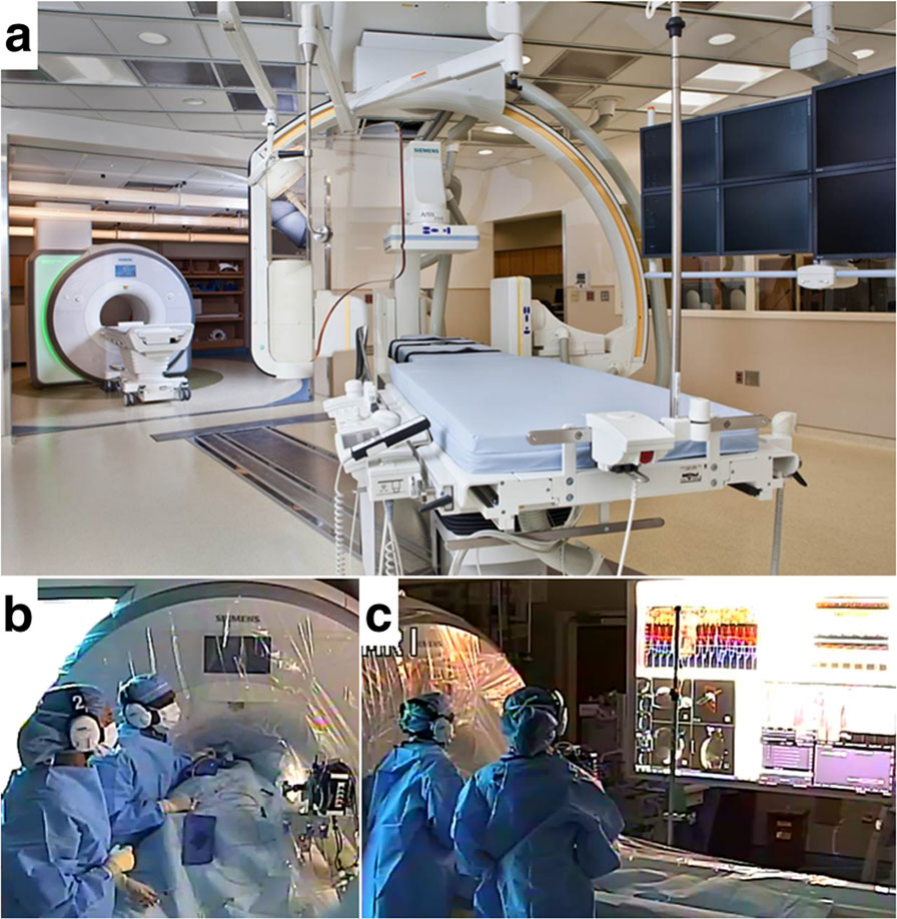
ICMR suite. CMR heart catheterization procedures were performed in an interventional CMR suite (Panel a) consisting of adjoining CMR room and biplane X-Ray fluoroscopy suite. The interventional cardiac MRI room is outfitted for invasive cardiac catheterization (Panels b, c). The patient and CMR scanner have sterile drapes. Operators observe sterile technique and wear noise-cancelling communication headsets. Commercially available passive catheters are connected to conventional pressure transducers that interface (panel b
